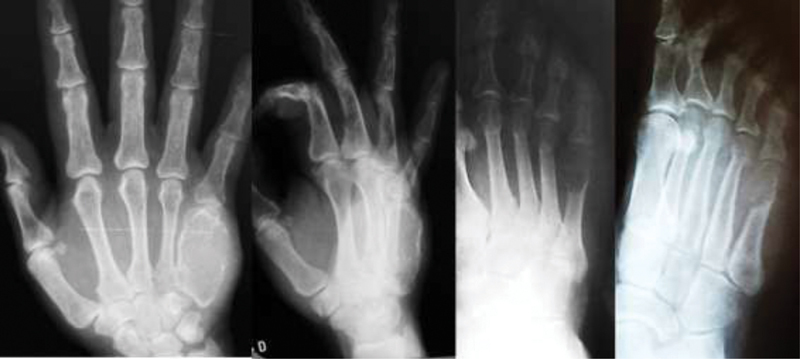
Case 2: right frontal and oblique radiographs of the right hand and foot, evidencing an expansive osteolytic lesion compromising the entire fifth right MC and the distal metaepiphyseal region of the fifth right metatarsus.
